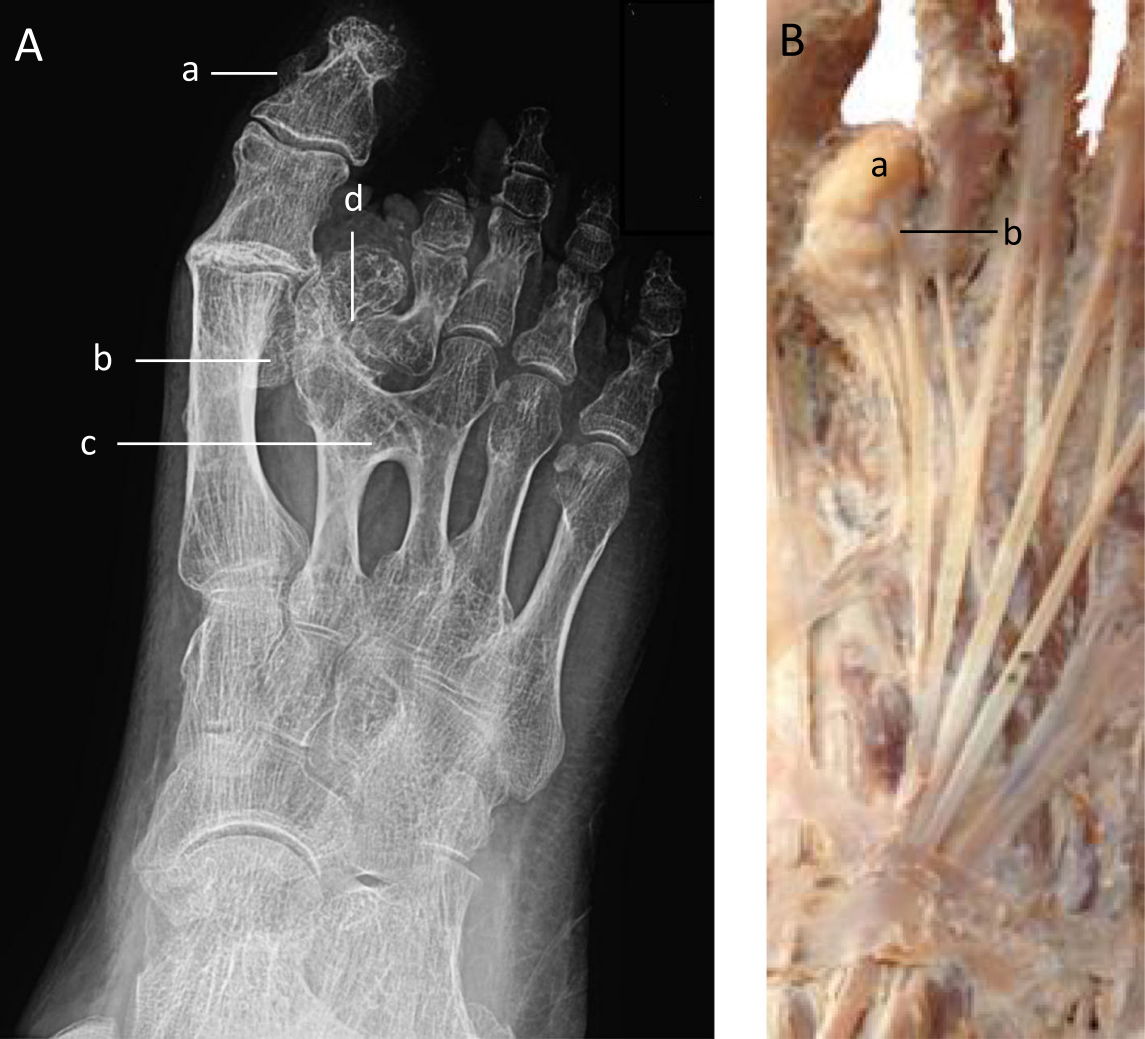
Right foot. a Radiograph. A pedunculated growth is present on the medial surface of the 1st distal phalanx (a). A sessile osteochondroma is situated on the inferior surface of the 1st metatarsal bone (b). A sysnostosis has formed between the heads of the 2nd and 3rd metatarsals (c). An osteochondroma of the 2nd metatarsal has altered the orientation of the metatarsophalangeal joint (d). b Superficial dissection. The cartilage capped osteochondroma can be seen at the head on the 2nd metatarsal (a). A tendon of extensor digitorum longus is observed inserting into the osteochondroma (b)
